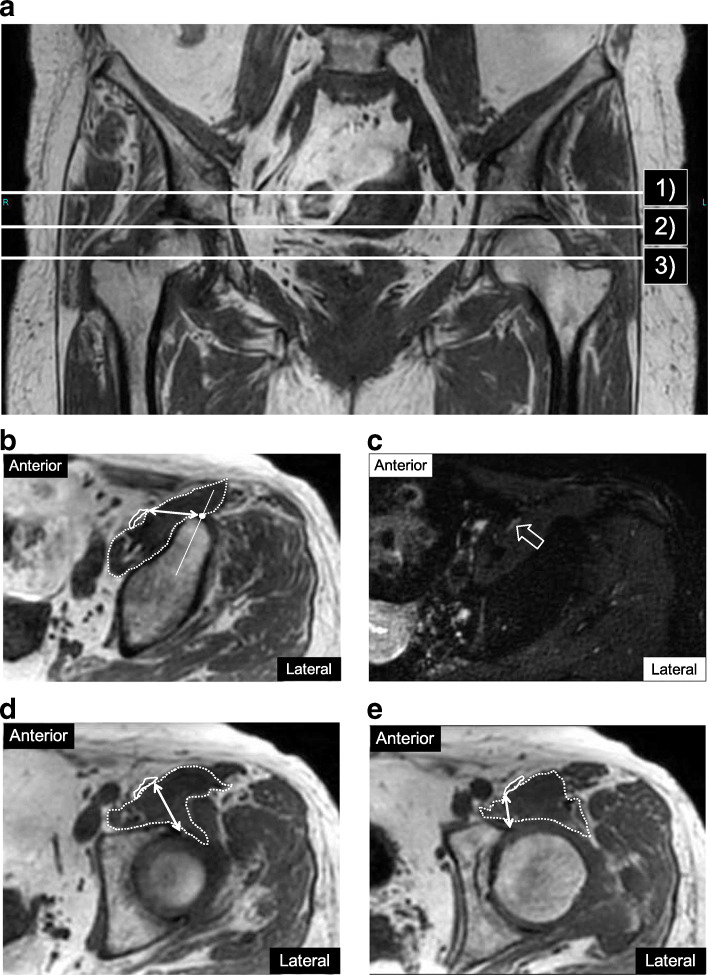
Magnetic resonance (MR) images showing measurements of the distance (white double-headed arrow) between the femoral nerve (FN) (solid line) and anterior acetabulum (AA), and the cross-sectional area (CSA) of the iliopsoas muscle (dotted line). a Measurement levels: (1) top of the anterior inferior iliac spine (AIIS), (2) bottom of the AIIS, (3) tip of the greater trochanter. b Axial T1-weighted image of the left acetabulum at level (1). The measurement point was defined as the intersection of the cortex and midline of the AIIS (white dot). The ambiguous FN was identified using the “nerve-sheath signal increased with inked rest-tissue rare imaging” (3D-NerveVIEW image. c Axial 3D-NerveVIEW image of the left acetabulum at the same level of B). The FN was clearly visualized in white (outline arrow). d Axial T1-weighted image of the left acetabulum at level (2). The measurement point on the acetabulum was defined as the head of the bony AA rim. The minimum FN to AA distance was measured from the lateral edge of the FN to the head of the acetabulum. e Axial T1-weighted image of the left acetabulum at level (3). The measurement point on the acetabulum was defined as the head of the bony AA rim. The minimum distance was measured from the medial edge of the FN to the head of the acetabulum
